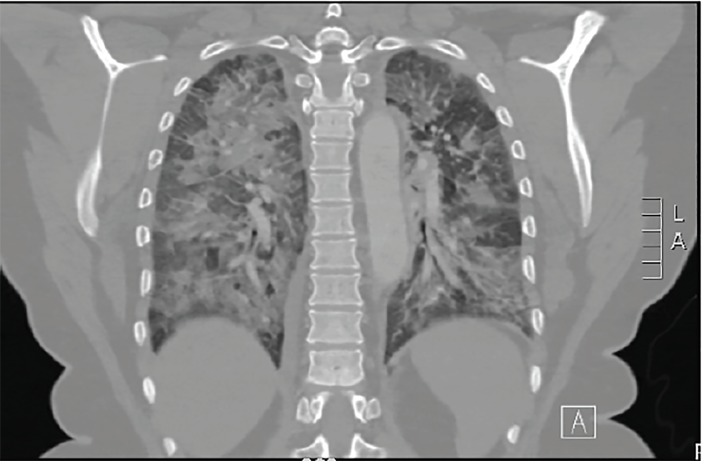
Coronal chest computed tomography demonstrated bilateral pulmonary edema.
